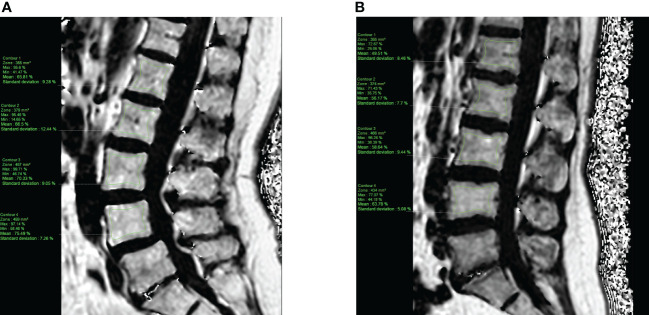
PDFF map of the lumbar spine computed from a T1-weighted multi-echo gradient echo sequence (mDixon-Quant) acquired in the sagittal plane, from a 63-year old control (A) and a 50-year old case (fragility fracture of the wrist, (B) post-menopausal women. Manually segmented ROIs (*green*) were placed in the vertebral bodies of L1 to L4, avoiding the immediate subchondral bone, the cortical bone and the basivertebral vein.
